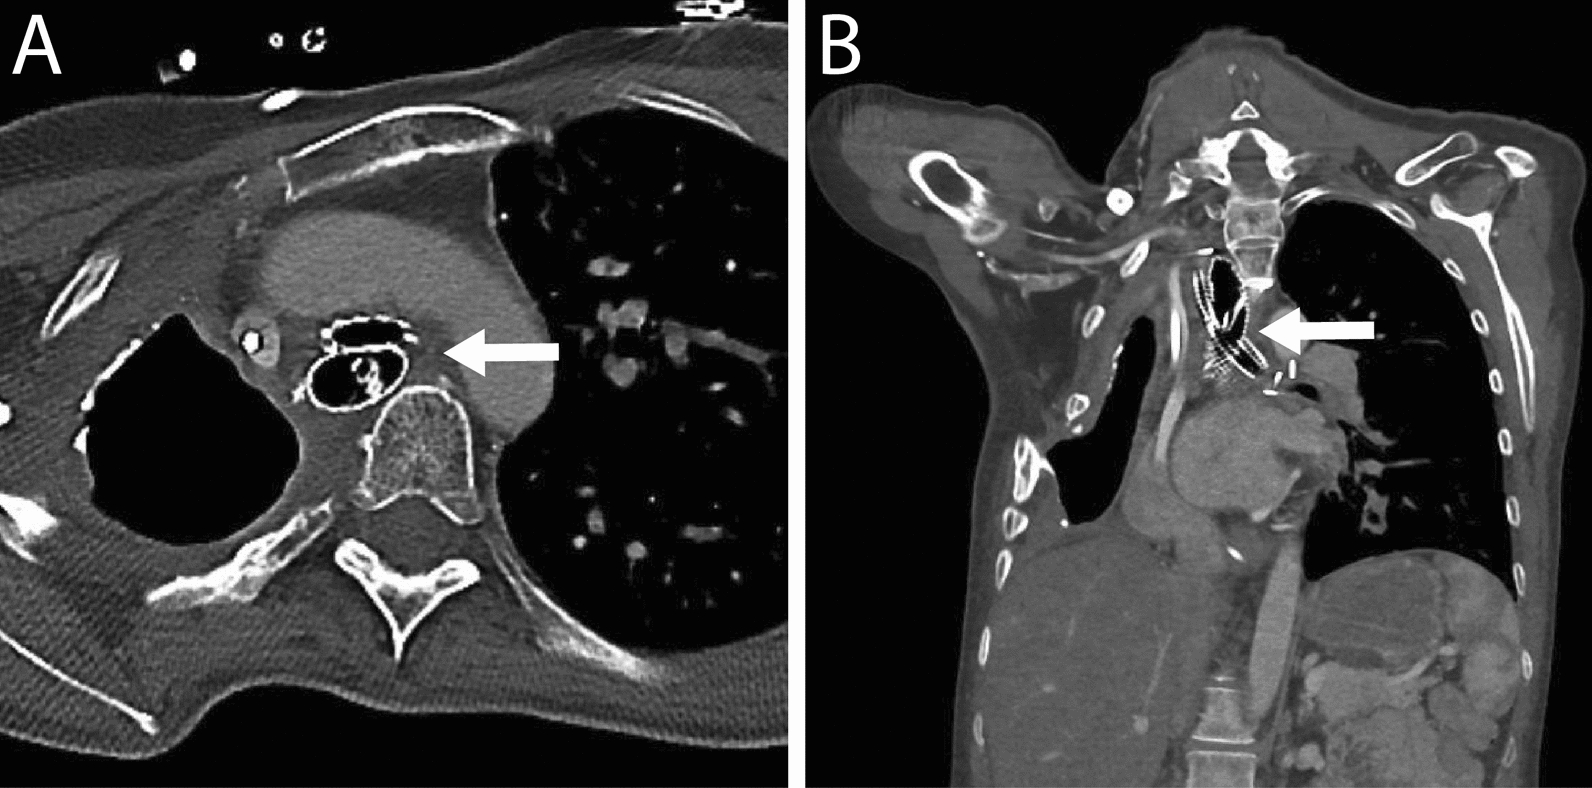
A–B. Chest CT scan showing locations and crossing of the esophageal and the tracheobronchial self-expanding covered metal stents (arrow)
